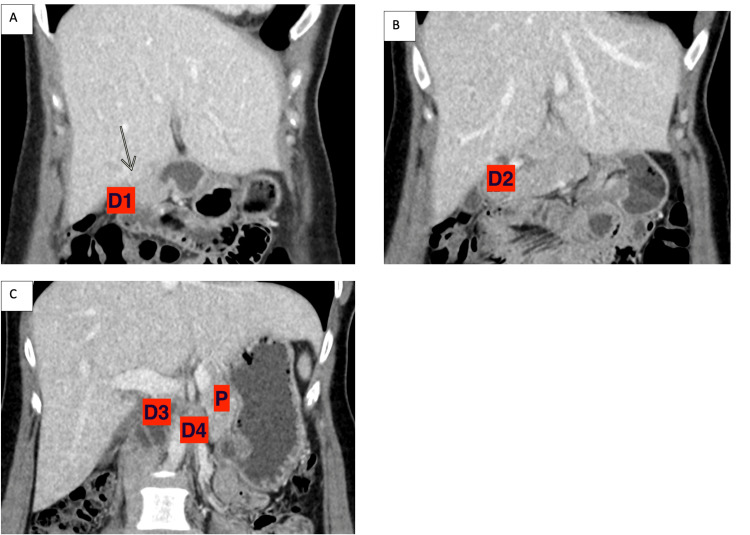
Contrast-enhanced CT of the abdomen showing the third part of the duodenum positioned higher relative to the second part and crossing the midline at the level of the pancreatic body (A) At the level of the first part of the duodenum (D1). (B) At the level of the second part of the duodenum (D2). (C) At the levels of the third part (D3) and fourth part (D4) of the duodenum.
